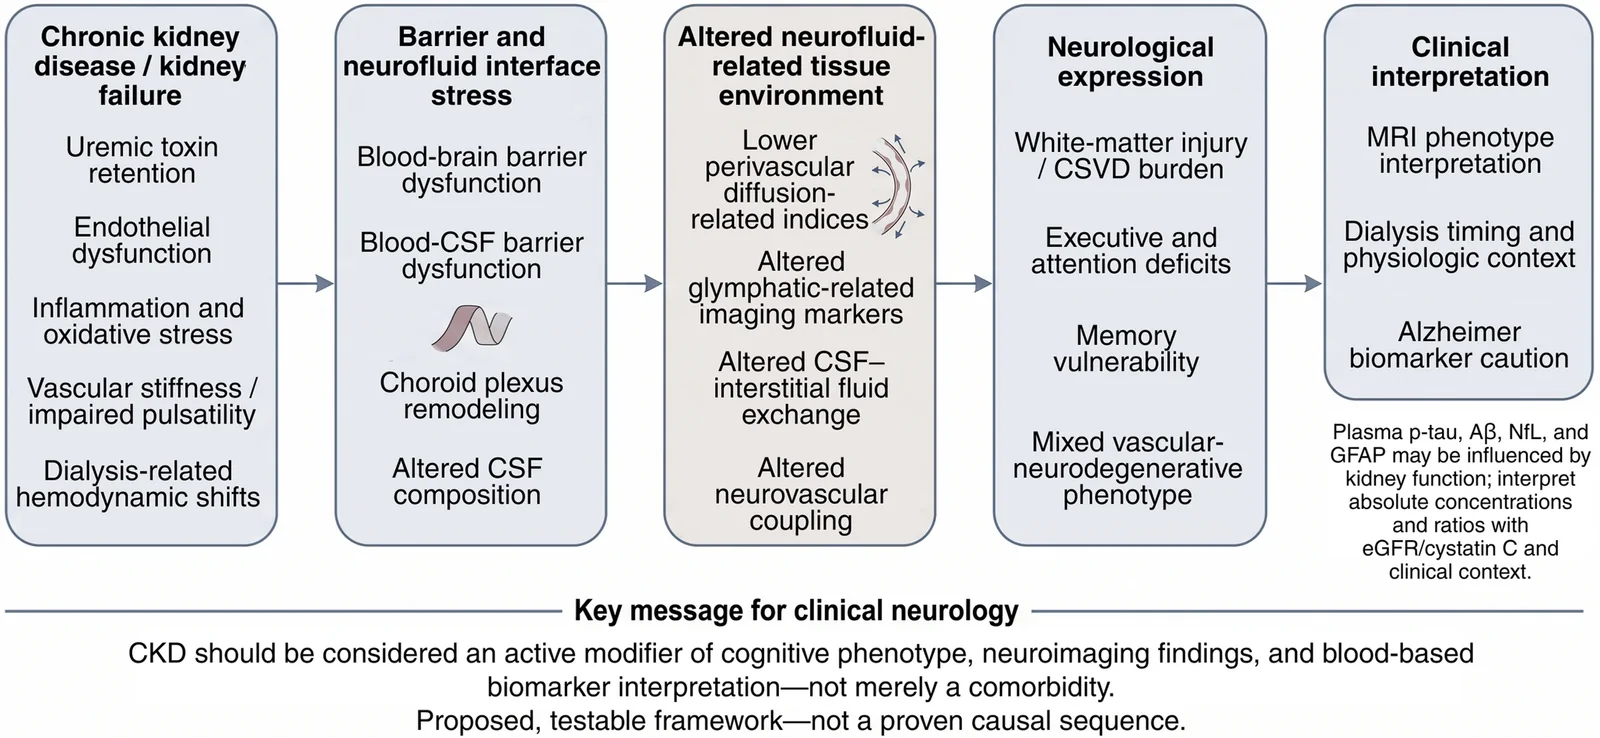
Proposed kidney–brain tissue-environment framework in chronic kidney disease. Chronic kidney disease and kidney failure may modify cognitive vulnerability through convergent systemic, vascular, barrier, neurofluid-related, white-matter, and biomarker-associated mechanisms. Uremic toxin retention, endothelial dysfunction, inflammation, oxidative stress, vascular stiffness, altered pulsatility, and dialysis-related hemodynamic or fluid shifts may coexist with blood–brain barrier dysfunction, blood–CSF interface stress, choroid plexus remodeling, and altered CSF composition. These processes may be associated with lower perivascular diffusion-related indices, altered glymphatic-related imaging markers, altered CSF–interstitial fluid exchange, and neurovascular dysregulation. The neurological phenotype may include white-matter injury, cerebral small-vessel disease, executive and attention deficits, memory vulnerability, and mixed vascular–neurodegenerative presentations. Plasma p-tau, amyloid-β, NfL, and GFAP concentrations may be influenced by kidney function and should be interpreted with eGFR, cystatin C, ratio-based measures where appropriate, and the broader clinical context. DTI-ALPS is a structure-dependent, ratio-based marker of localized directional diffusivity and not a direct measurement of glymphatic flow. Arrows indicate hypothesized, non-exclusive associations and should not be interpreted as temporal ordering, causal mediation, or a diagnostic algorithm. Abbreviations: Aβ, amyloid-β; CKD, chronic kidney disease; CSF, cerebrospinal fluid; CSVD, cerebral small vessel disease; DTI-ALPS, diffusion tensor imaging analysis along the perivascular space; eGFR, estimated glomerular filtration rate; GFAP, glial fibrillary acidic protein; MRI, magnetic resonance imaging; NfL, neurofilament light chain; p-tau, phosphorylated tau
